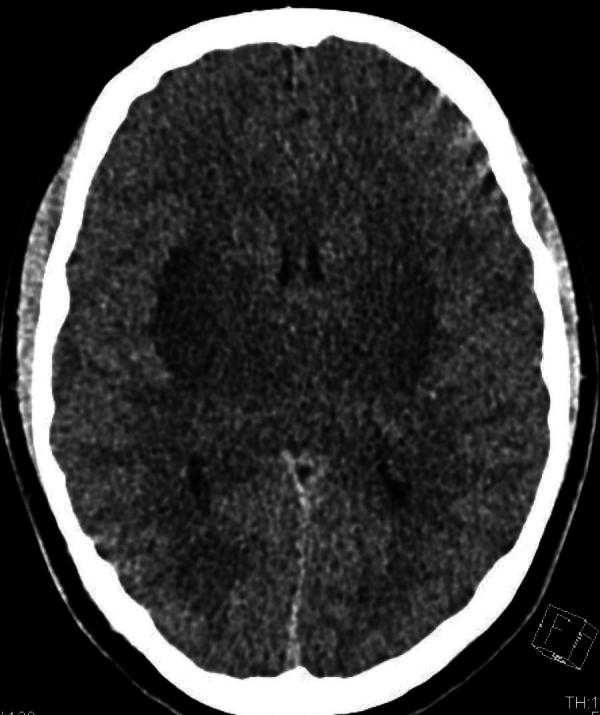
Axial CT scan showing hypodense lentiform nuclei and thalami.
